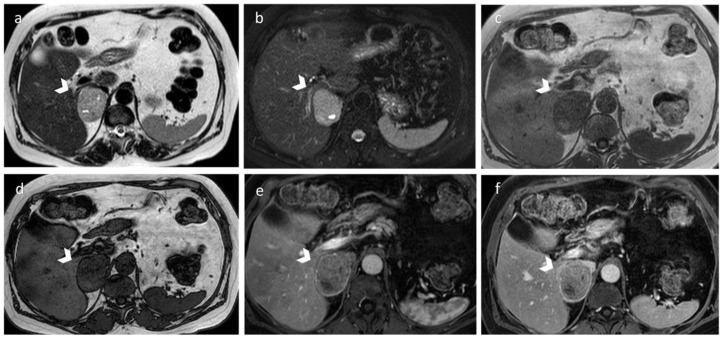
A 65-year-old female with right adrenal pheochromocytoma. MRI revealed a 5 cm solid lesion characterized by intermediate T2 signal (a,b) and no T1 signal drop in T1 out-of-phase sequences (c,d). Post-contrast acquisitions in arterial and portal venous phase (e,f) reveal inhomogeneous signal intensity and necrotic components.
